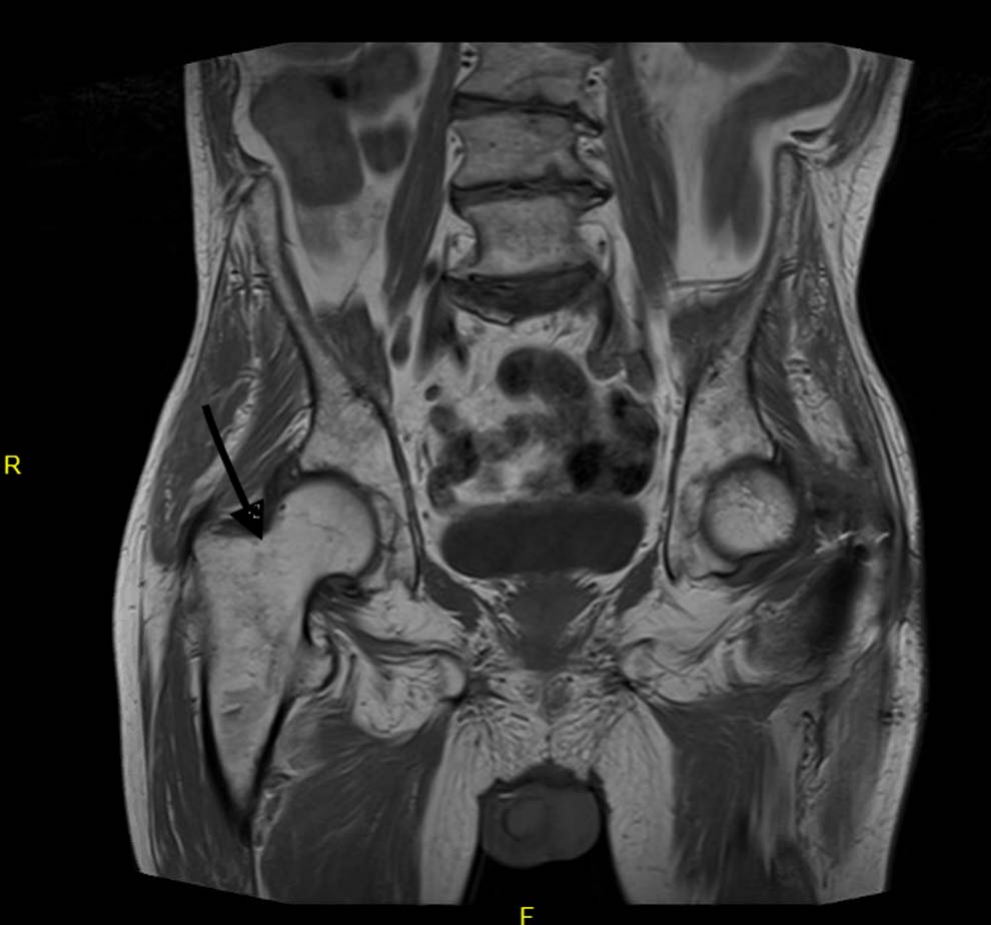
T1 magnetic resonance imaging of the pelvis shows a right greater trochanteric fracture with extension into the lesser trochanter.
